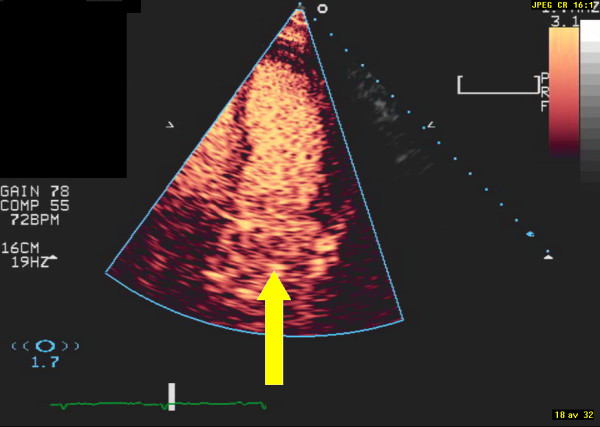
Image from the apical 4-chamber view after contrast injection. The arrow indicates what appears to be irregular contrast uptake within the structure in the left atrium.
